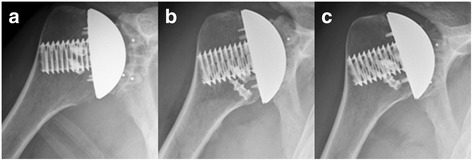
Anteroposterior radiographs of a forty-year-old man made immediately after total shoulder arthroplasty (a), at the short-term showing partial osteolysis at the inferior trunion bone interface (b), and at the mid-term follow-up showing stable partial inferior osteolysis and minor superior radiolucency (c)
